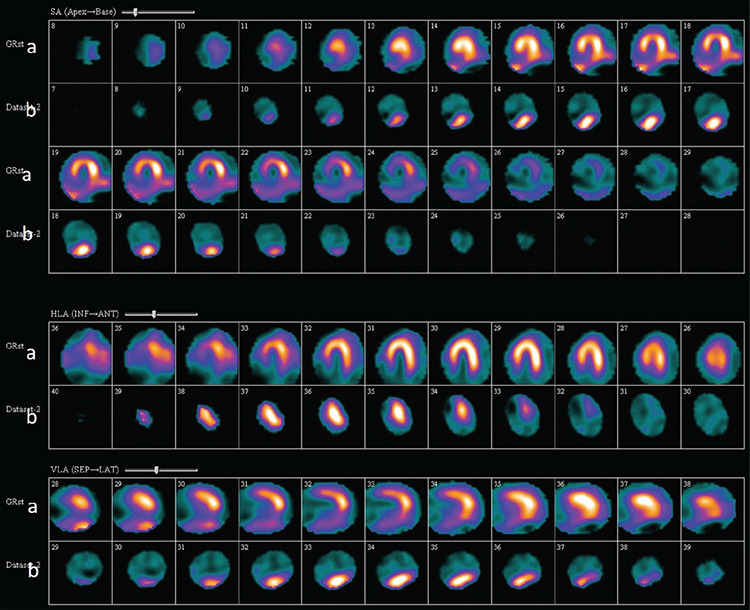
(a) Myocardial perfusion imaging using Tc-99m-MIBI SPECT at rest in a 51-year-old man. Short, vertical, and horizontal slices in the top rows, showing severely decreased uptake in the inferior and inferoseptal walls. (b) Myocardial SSTR imaging with Tc-99m labeled octreotide SPECT in the same patient. Short axis, vertical, and horizontal slices in the lower rows showed uptake in the inferior region suggesting vulnerable plaque.
